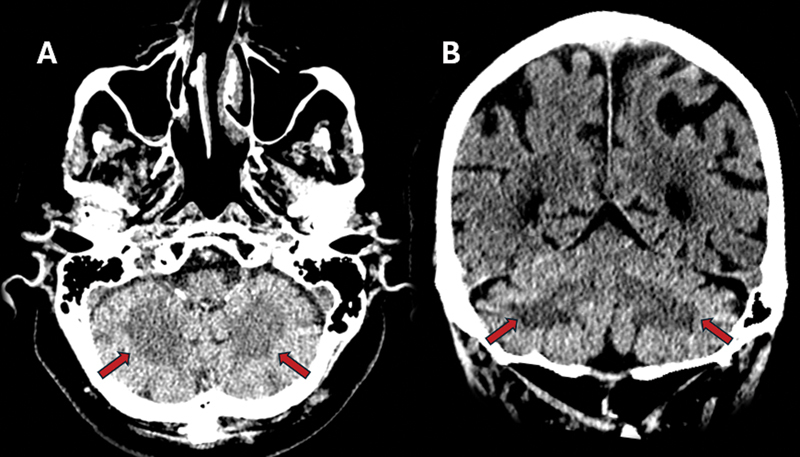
Non-contrast brain computed tomography (CT) scan, axial (
